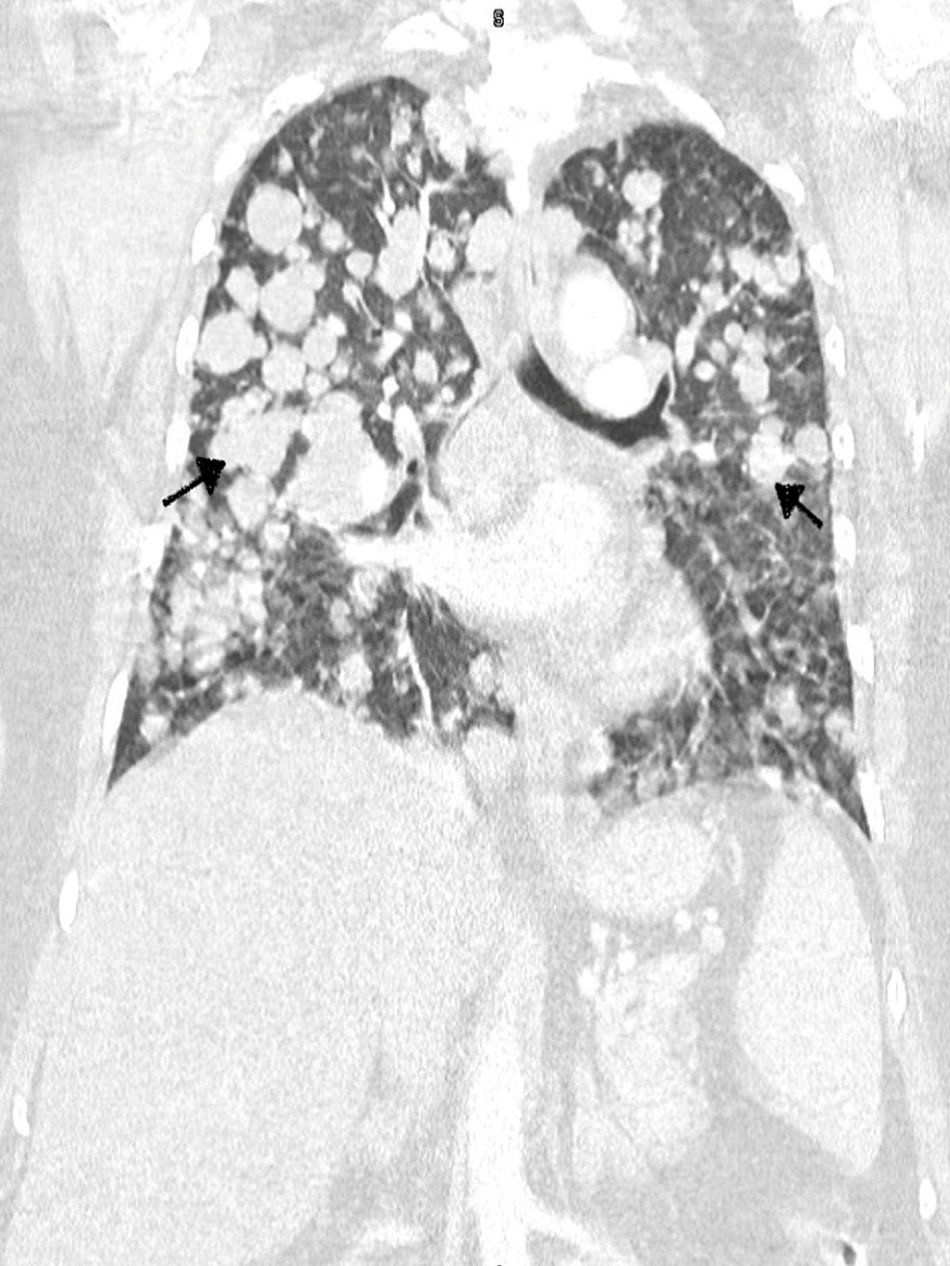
CT chest showing multiple lesions in the lung suggestive of metastasis (black arrows)
